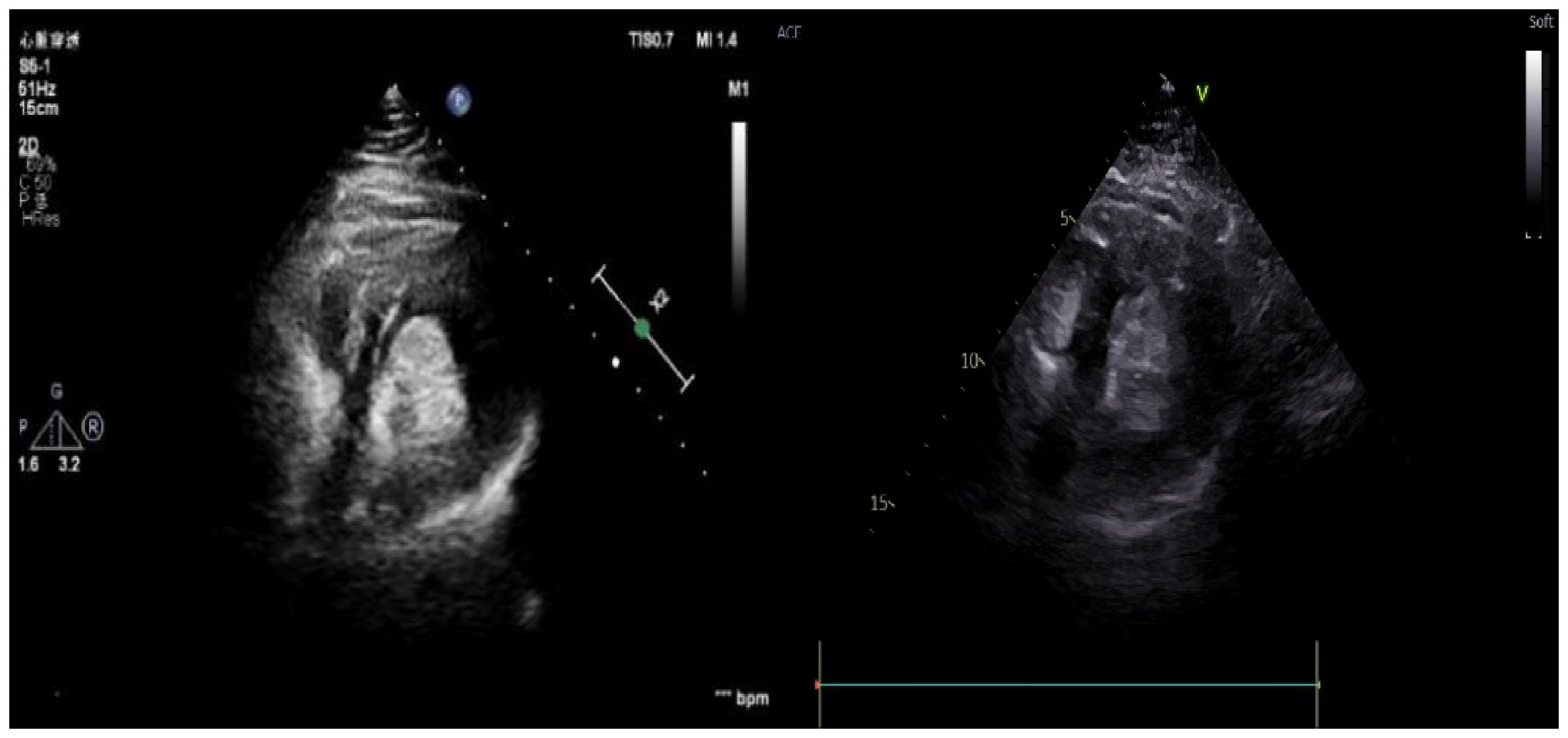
Contrast-enhanced ultrasound results.
